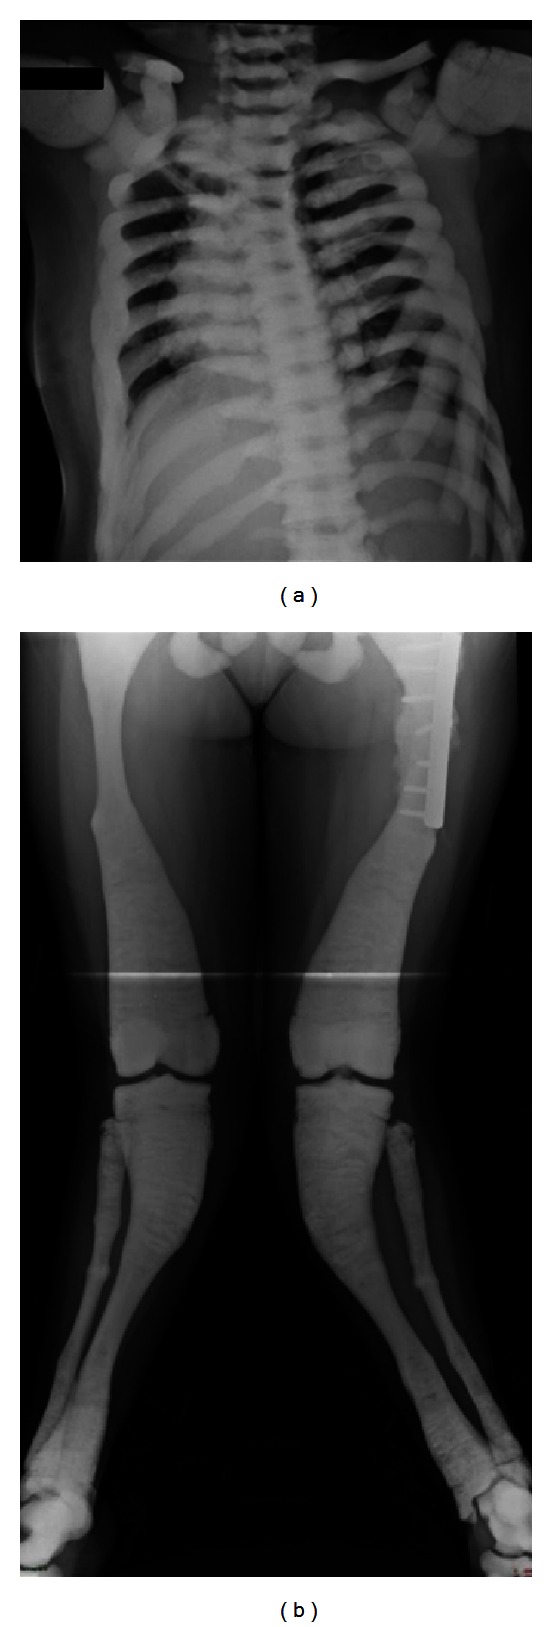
X-rays of patient S7 at the last followup showing right scoliotic deviation of the dorsal spine, knee valgus, and deviation of the tibia with multiple fractures of the tibia and fibula.
